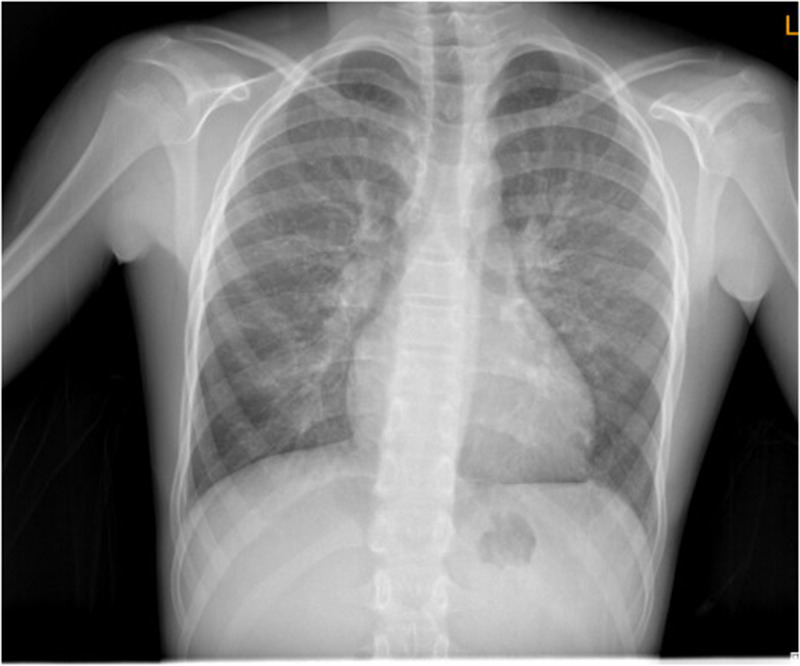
Posteroanterior (PA) chest radiograph demonstrating bilateral pulmonary infiltrates. The image shows diffuse opacities in both lung fields, consistent with pulmonary edema. Pulmonary vascular congestion is apparent. These findings correlate with the patient's acute hypoxic respiratory failure and were key in prompting further cardiac evaluation, including transthoracic echocardiography, which revealed cor triatriatum sinister. No focal consolidation or pleural effusion is noted, helping to differentiate cardiogenic pulmonary edema from primary respiratory infection.
